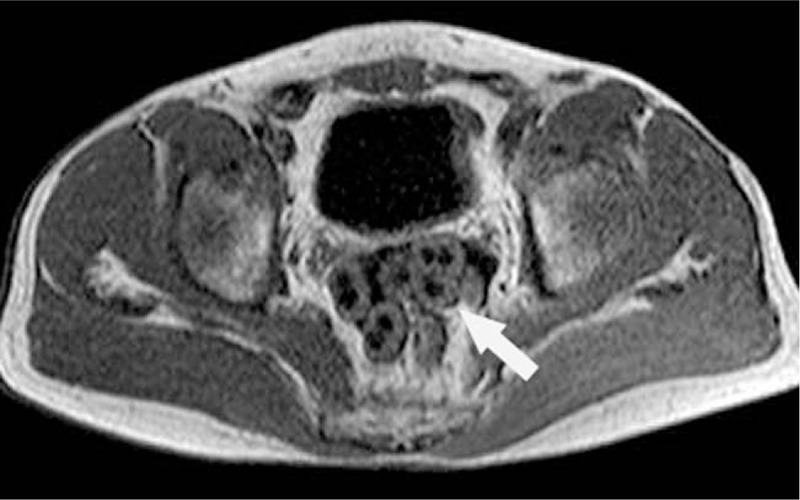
MR images show multiple abnormal nodules in rectovesical pouch. T1WI reveals central hypointensity and peripheral ring-like isointensity (arrow), T2WI reveals homogeneously hypointensity (arrow). MR = magnetic resonance.
